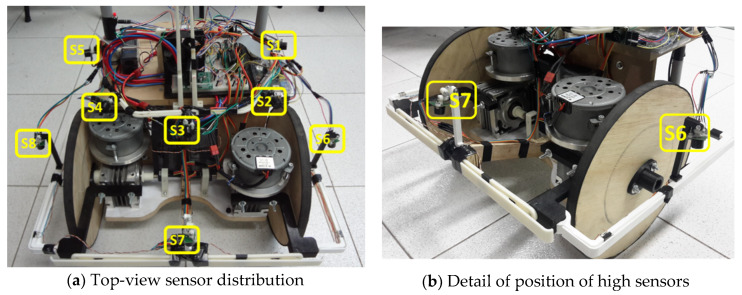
Distribution of ultrasonic sensor at the prototype.
